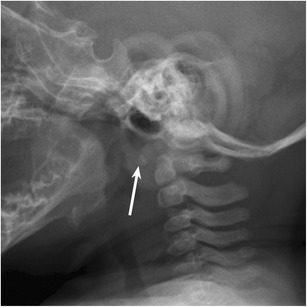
Normal lateral radiograph of the cervical spine in a 13 month old reveals the ossification centre of the body of the atlas. The posterior arches have not yet appeared
